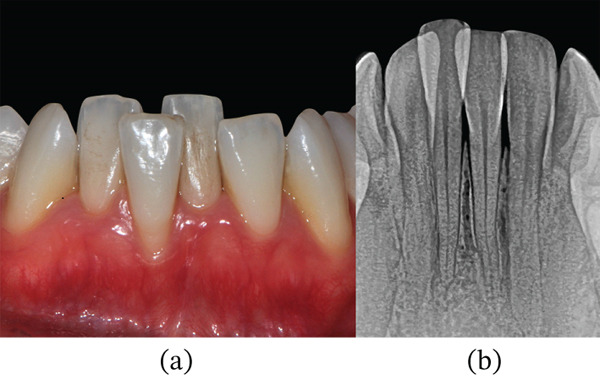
Baseline: (a) clinical and (b) radiographic view.
